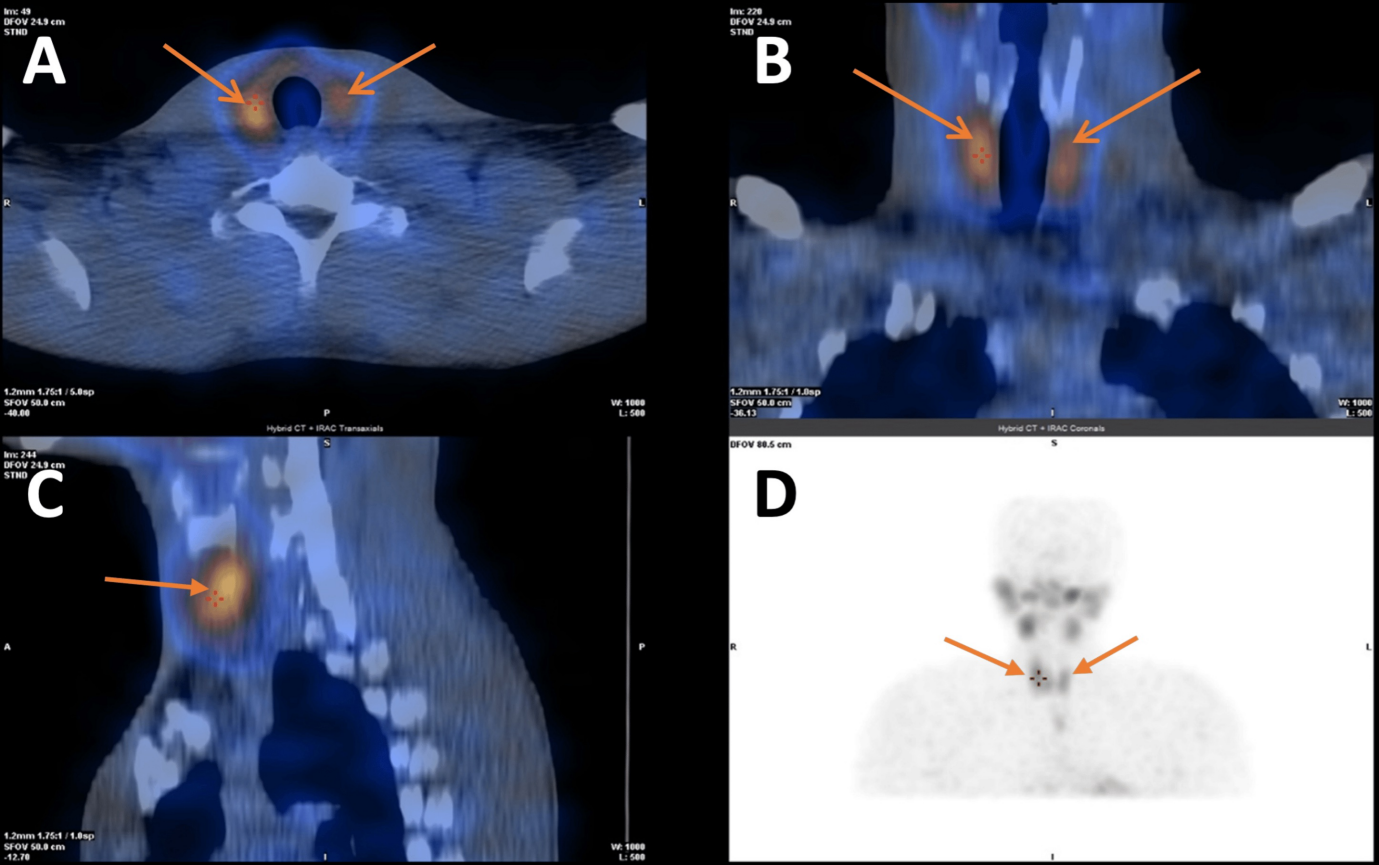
Parathyroid dual isotope (I-123 and Tc-99m-sestamibi) planar pinhole subtraction scintigraphy with subtraction SPECT/CT demonstrating reactive secondary hyperparathyroidism and excluding a primary parathyroid adenoma (A) Axial dual isotope subtraction SPECT/CT showing reactive parathyroid glands bilaterally with the right glands being more reactive than the left (arrows). (B) Coronal dual isotope subtraction SPECT/CT showing reactive parathyroid glands bilaterally with the right glands being more reactive than the left (arrows). (C) Sagittal dual isotope subtraction SPECT/CT showing a reactive parathyroid gland (arrows). (D) Coronal dual isotope planar pinhole subtraction scintigraphy showing reactive parathyroid glands bilaterally with the right glands being more reactive than the left (arrows) SPECT/CT: single-photon emission computed tomography/computed tomography
